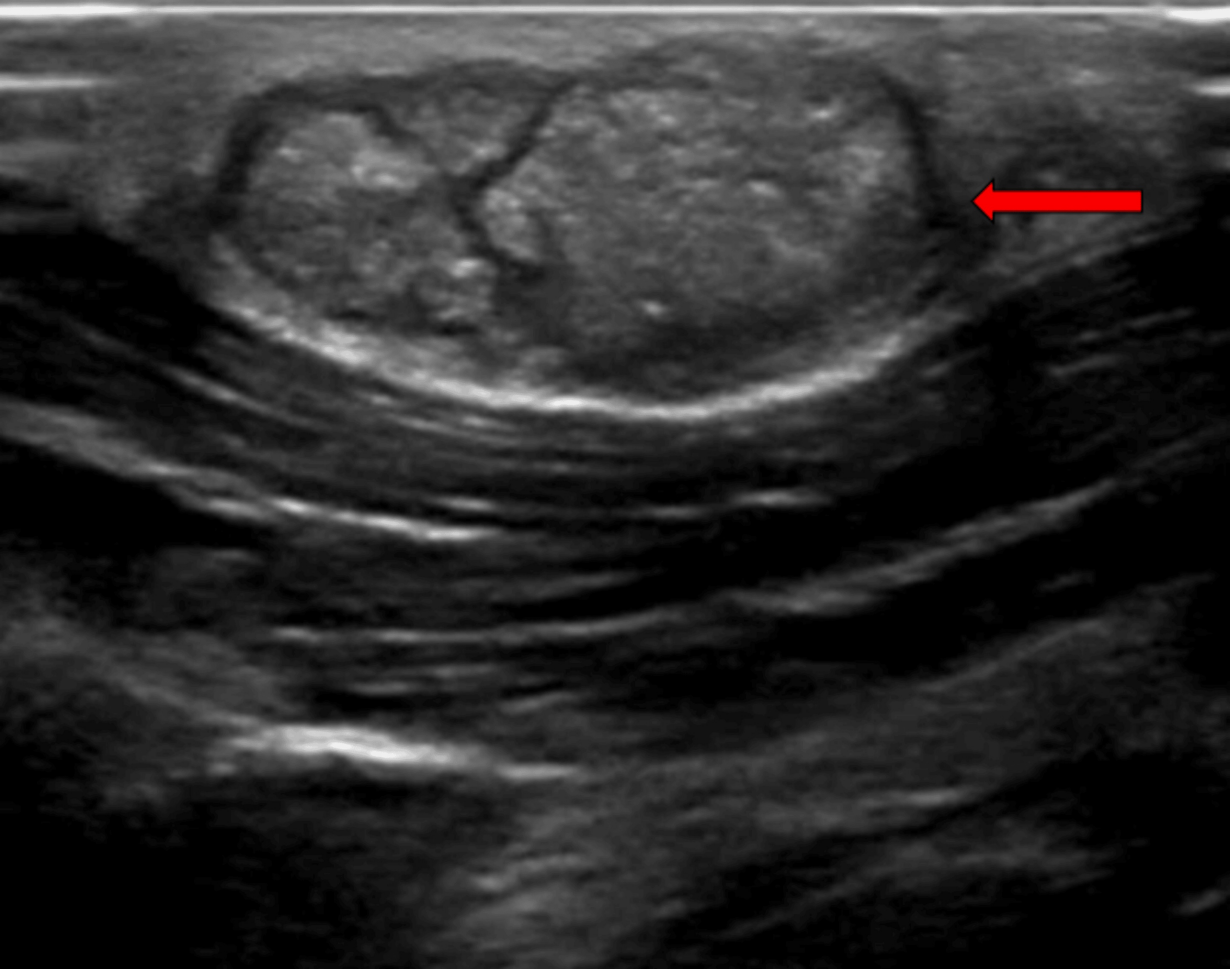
Ultrasonography of the local region (red arrow) showing a single well-defined encapsulated lesion with proteinaceous material within the subcutaneous plane.
